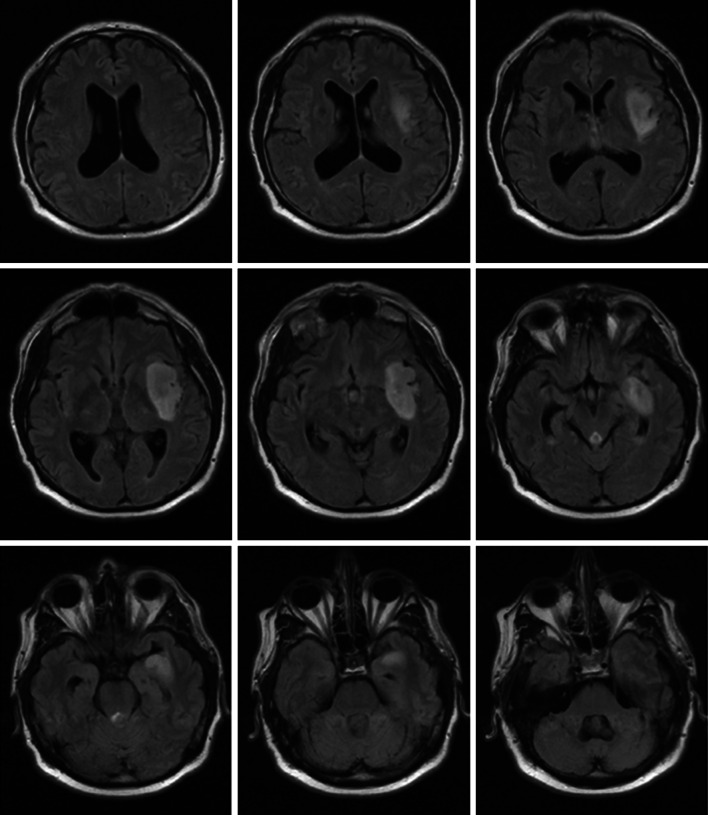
How would you treat this patient? A 49 year old male right hand dominant presents with complex-focal seizures, without any neurological deficit. MRI FLAIR sequence is shown. There was no enhancement with gadolinium
